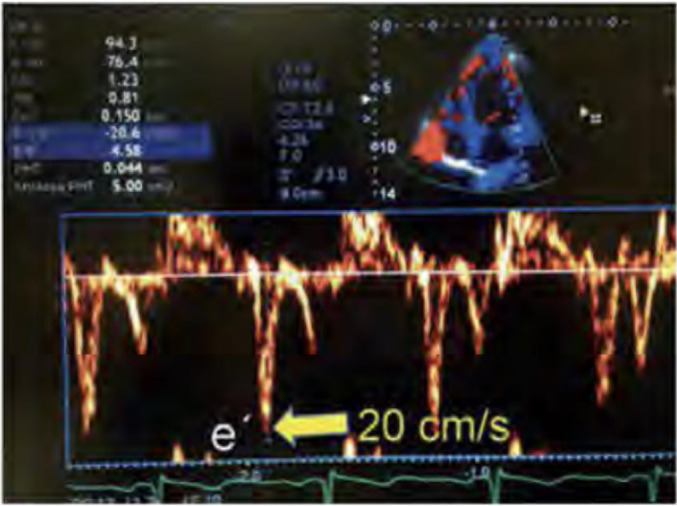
Tissue Doppler ultrasound of the basal lateral wall of the left
